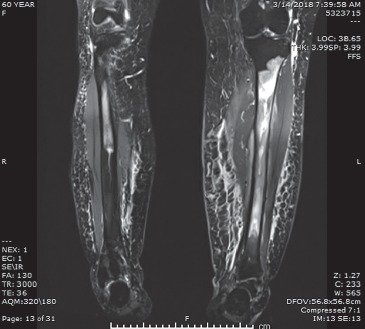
MRI of left tibia with/without IV contrast.
